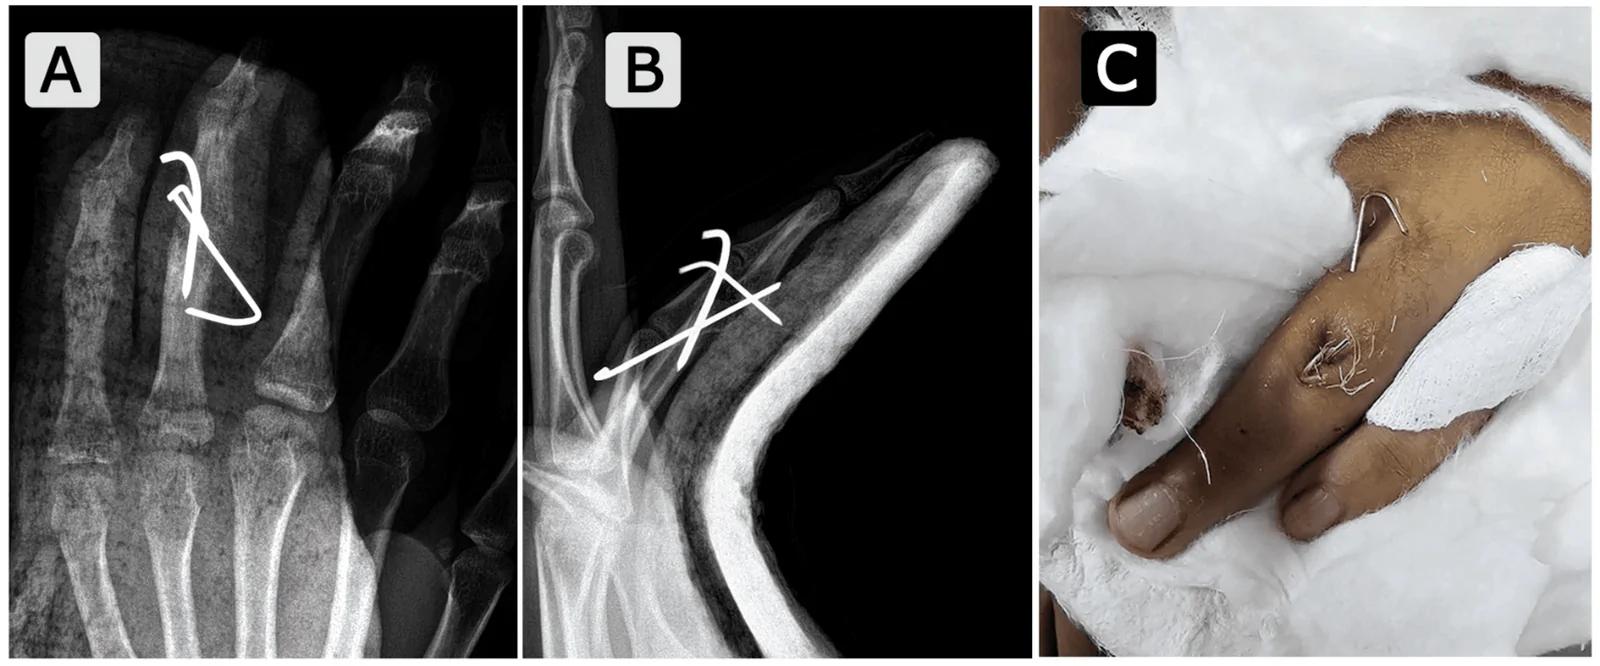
Early postoperative follow-up (13 days post-surgery). (A) AP and (B) lateral radiographs confirming maintained concentric reduction with the three K-wires in situ: extension block K-wire (1.5 mm), fragment fixation K-wire (1.2 mm), and transarticular stabilization K-wire (1.2 mm). (C) Clinical photograph demonstrating the K-wire configuration with the PIP joint maintained in full extension AP: anteroposterior
